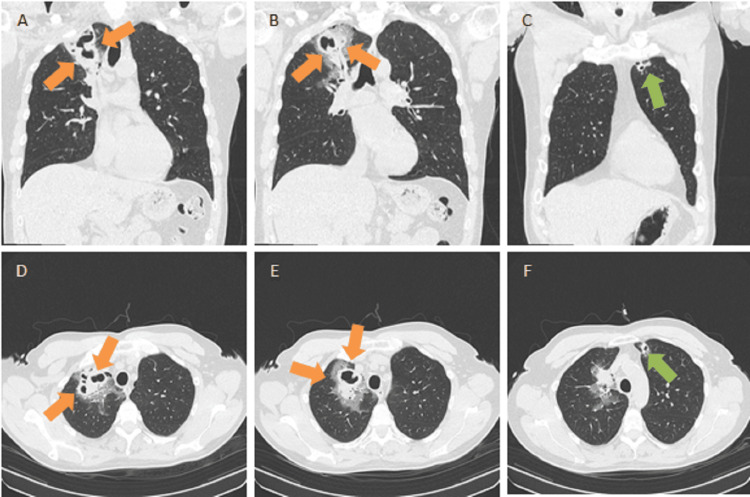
Images of the thoracic computed tomography performed at admission (A and B) Coronal views showing partially cavitated lesions in the right upper lobe (orange arrows). (C) Coronal view showing cavitated lesion in the left upper lobe (green arrow). (D and E) Axial views showing partially cavitated lesions in the right upper lobe (orange arrows). (F) Axial view showing cavitated lesion in the left upper lobe (green arrow)
